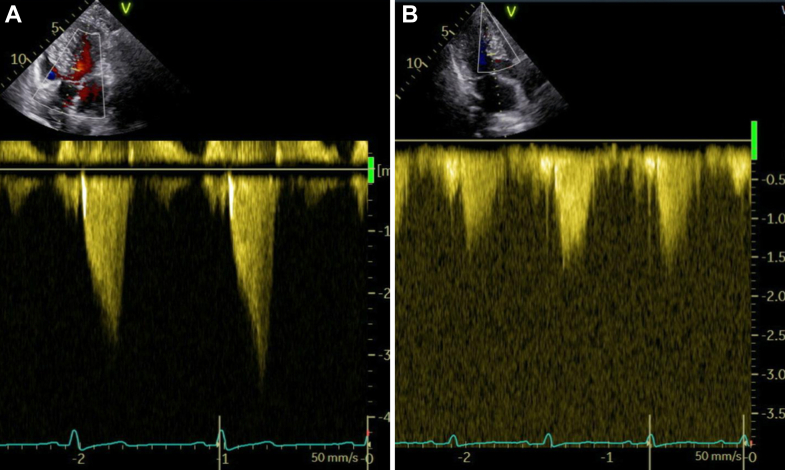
Baseline and Follow-Up LVOT Gradients on Transthoracic Echocardiography LVOT gradient during the Valsalva maneuver (A) at baseline and (B) at the 6-month follow-up. LVOT = left ventricular outflow tract.
